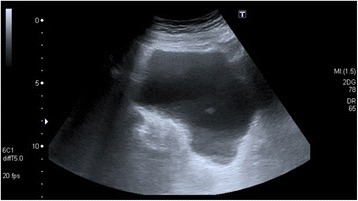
Ultrasound of the bladder demonstrating irregular thickening of the bladder wall with extensive intramural echogenic foci.
